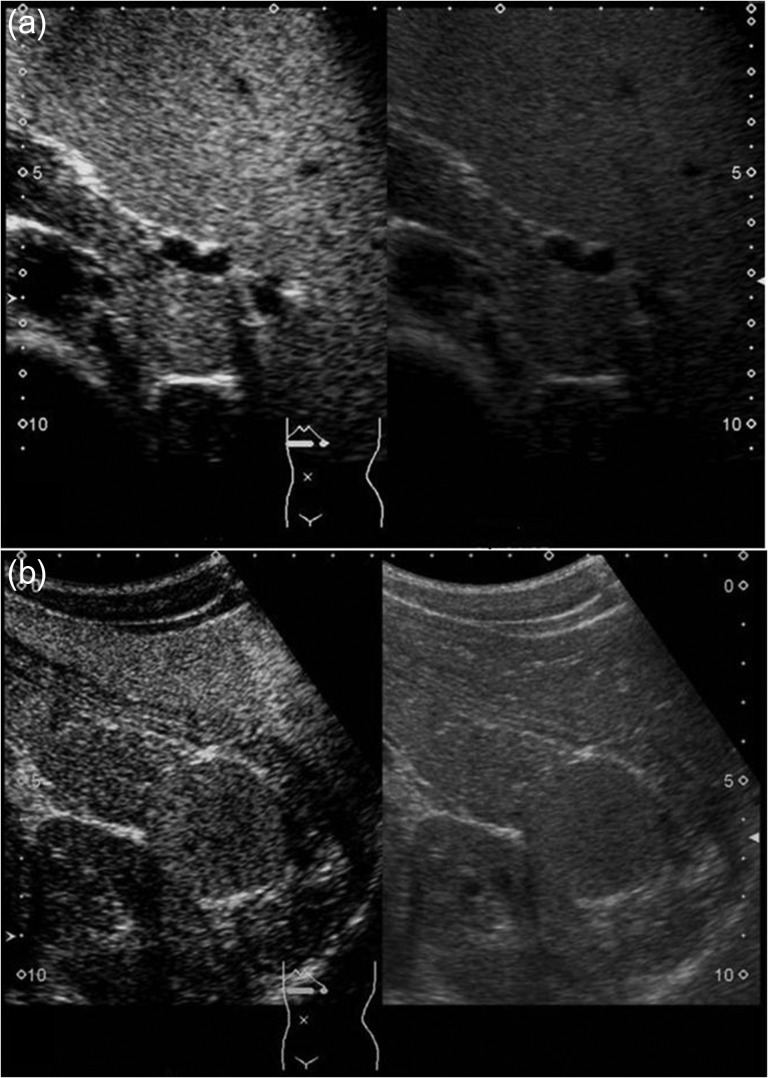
Sonazoid-enhanced ultrasonography Presplenectomy enhanced ultrasonography shows hypervascularity in the mass, located near the pancreatic tail and suspicious for an accessory spleen. The mass grows rapidly to 4 cm by 1 year after splenectomy (a: before splenectomy, b: after splenectomy).
